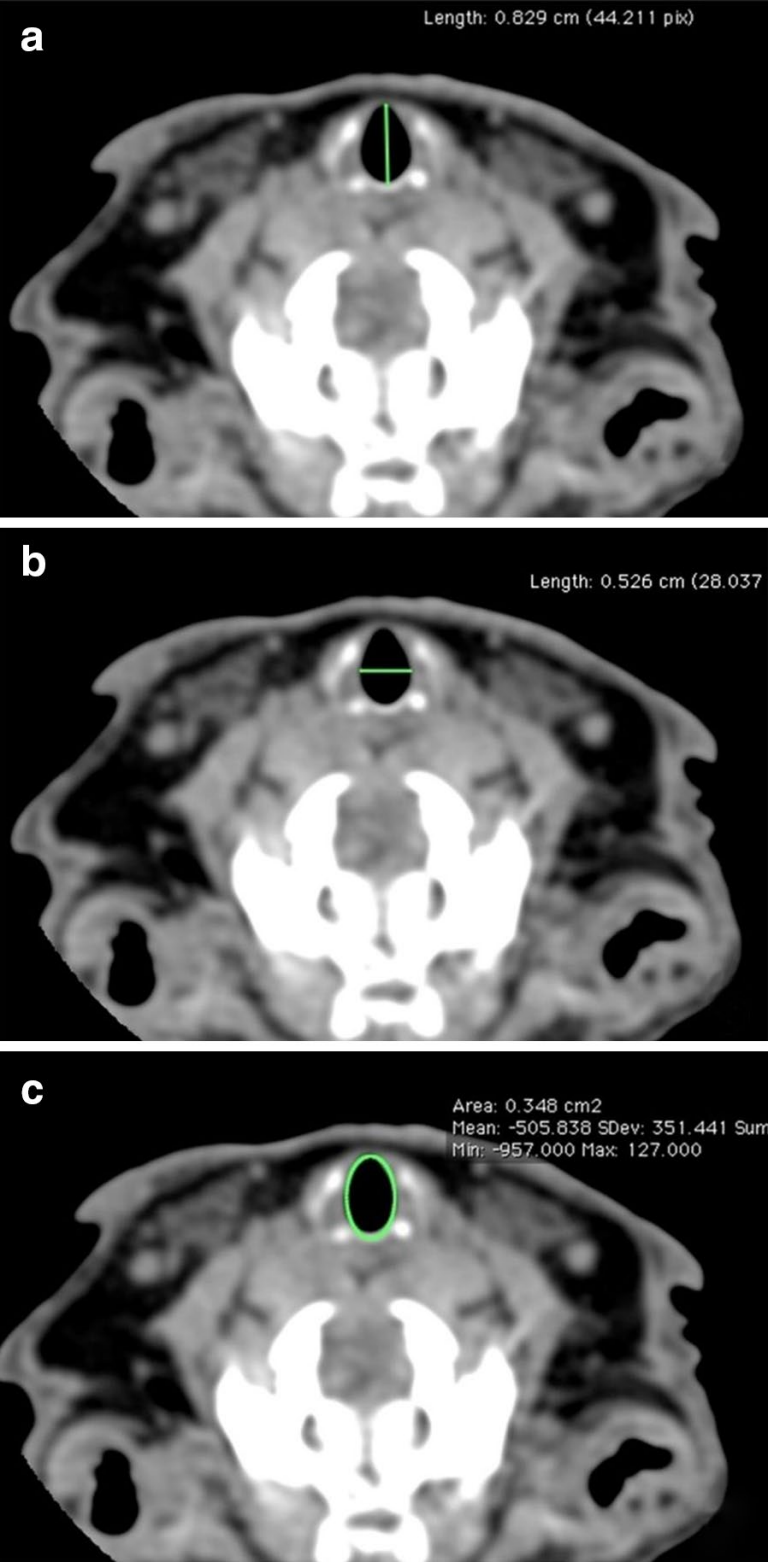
Representative images of computed tomography measurements used in this study. a Transverse CT image showing the trachea with an anteroposterior diameter of 8.3 mm at the arytenoid cartilage level. b Transverse CT image showing the trachea with a transverse diameter of 5.3 mm at the arytenoid cartilage level. c Transverse CT image showing the trachea with an area of 34.6 mm^2^ at the arytenoid cartilage level
